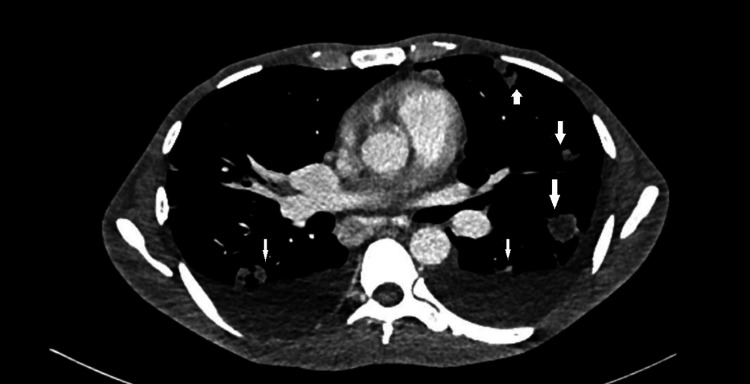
CT chest image showing bilateral small lung abscesses with pleural effusion
